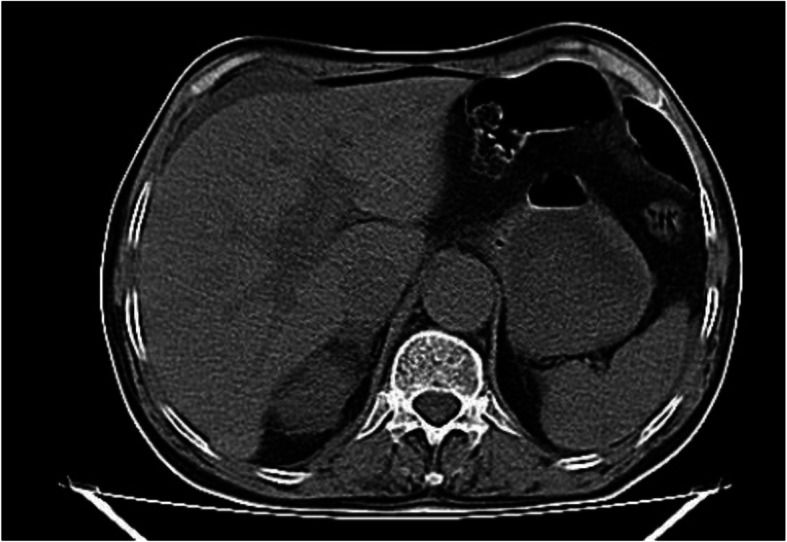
Abdomen view in Primary CT scan. A small amount of perihepatic and perisplenic fluid was observed on the patient’s CT scan
